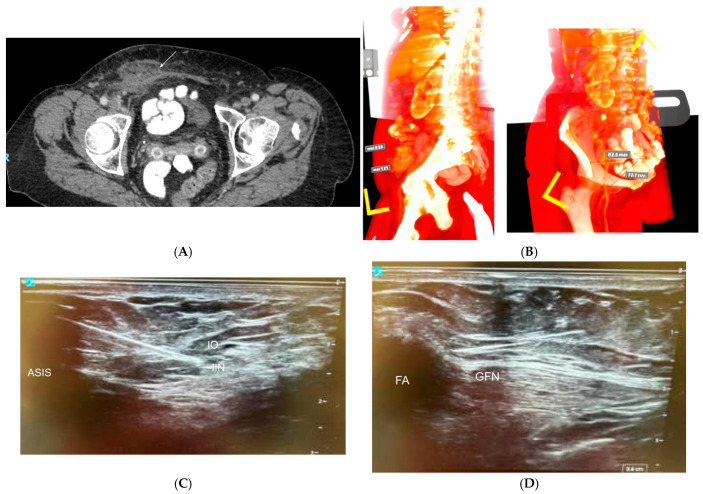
Patient 5, who underwent 2-lead PNS implantation for chronic abdominal wall pain. (A) CT scan demonstrating seroma adjacent to prior right inguinal hernia repair (white arrow). Neurectomy of iliohypogastric nerve also occurred during this operation. (B) Volumetric reconstruction of patient CT abdomen, utilized to plan the best path for lead deployment prior to the operation. (C) Ultrasound-guided ilioinguinal/iliohypogastric nerve PNS deployment. ASIS = anterior superior iliac spine, IIN = ilioinguinal nerve, IO = internal oblique muscle. (D) Ultrasound-guided genitofemoral nerve PNS deployment. GFN = genitofemoral nerve, FA = femoral artery.
